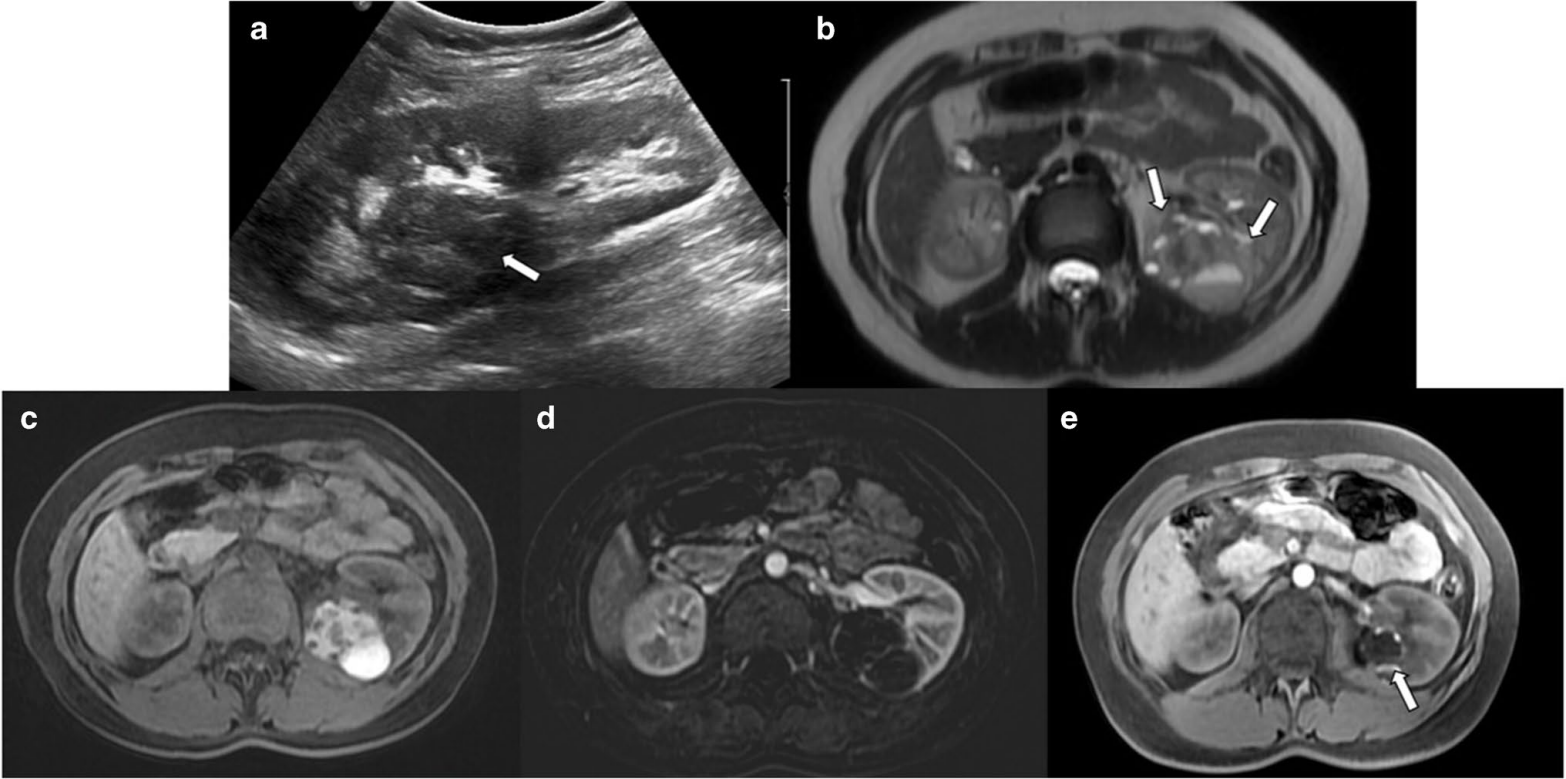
Hemorrhagic cyst. A 17-year-old boy was admitted to the Emergency Department with sudden onset left flank pain. a Longitudinal grayscale ultrasound image of the left kidney demonstrates a heterogeneous lesion (*arrow*) extending from the renal sinus to the capsule. b Axial T2-weighted image shows a lesion containing some cystic areas and fluid–fluid level (*arrows*) in the left kidney. c Axial fat-suppressed pre-contrast T1-weighted image reveals that the lesion is predominantly hyperintense, consistent with hemorrhagic content. d Axial post-contrast substraction image demonstrates only septal enhancement without any solid component, consistent with hemorrhagic cyst. e Follow-up axial fat-suppressed pre-contrast T1-weighted image obtained 3 months later shows a decrease in the lesion size and regression of most of the hemorrhagic content (*arrow*)
